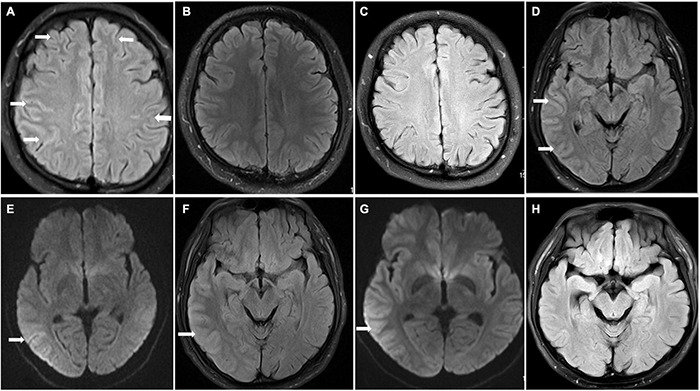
Brain magnetic resonance imaging (MRI) findings in patients 1 and 2. Patient 1 (A–C) On admission, FLAIR hyperintensity was seen in the leptomeningeal of bilateral frontal lobe and parietal lobe in patient 1 (A) (arrowhead); repeat brain MRI FLAIR result returned to normal at discharge and 8 months after discharge (B,C). Patient 2 (D–G) Brain MRI results showed T2 FLAIR and DWI hyperintensity (D,E) (arrowhead) with swelling of brain tissue and narrowing of sulcus; the swelling of brain tissue was alleviated, and hyperintensity of FLAIR and DWI was improved after immunotherapy (F,G) (arrowhead); brain MRI normalized at 1 month after discharge (H).
